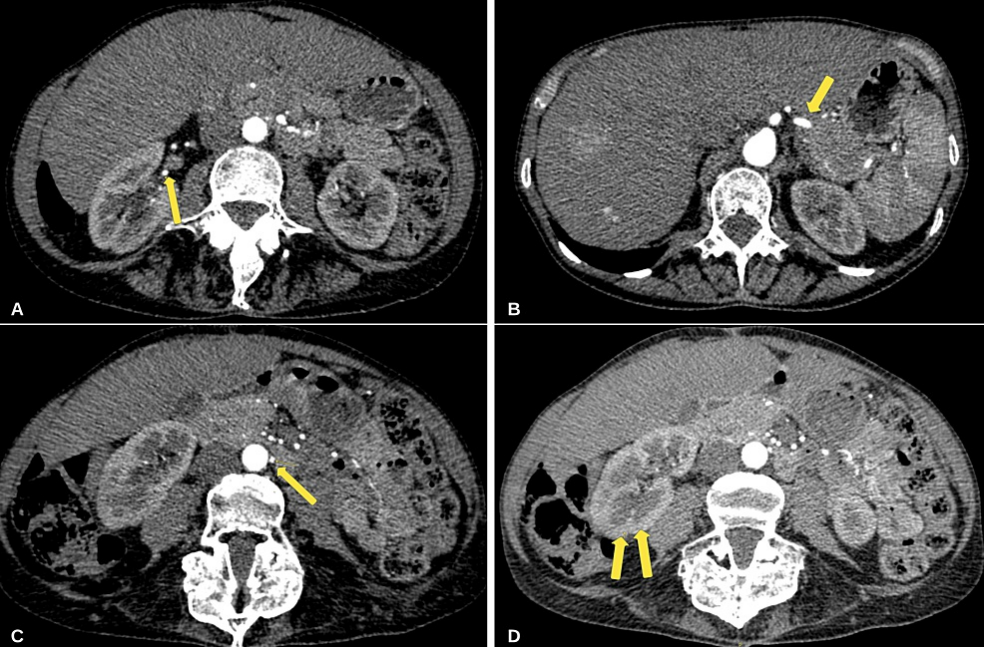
Repeat CT abdomen and pelvis angiography two months after discharge. (A-C) Resolution of prior vascular irregularities of the left renal, splenic, and inferior mesenteric arteries. (D) Resolution of renal parenchymal hypo-enhancement.
